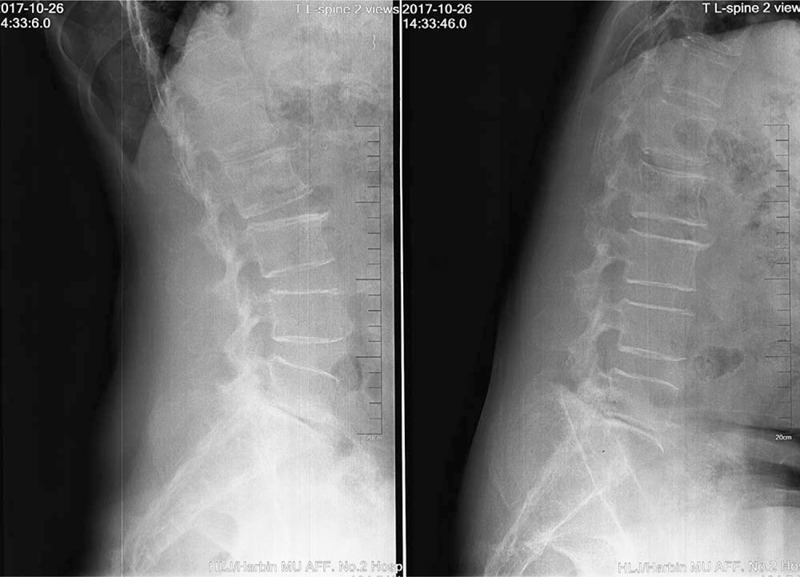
Radiographs showing obvious osteoporosis in the vertebrae and osteosclerosis in the endplates of L2, L3, and L4. Osteosclerosis was also found in the upper posterior half of the L2 vertebral body.
